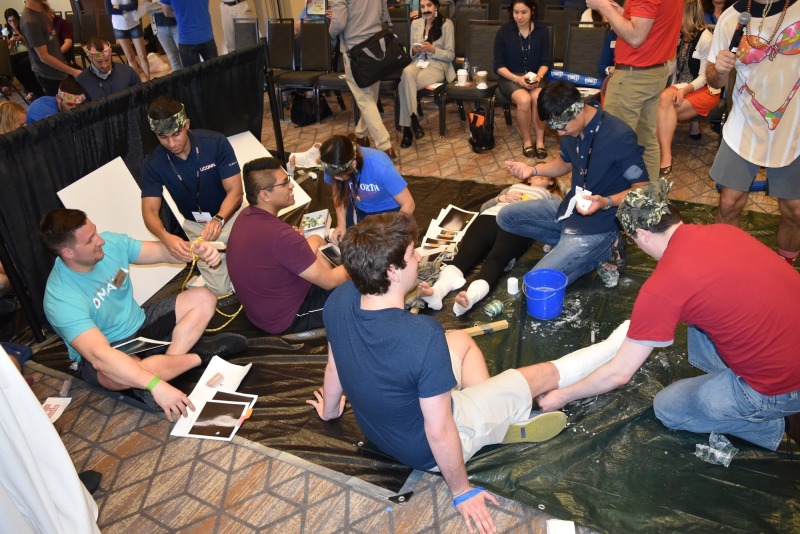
Participants are seen splinting a broken leg while matching X-ray images to injured patients as part of Round 1A.
